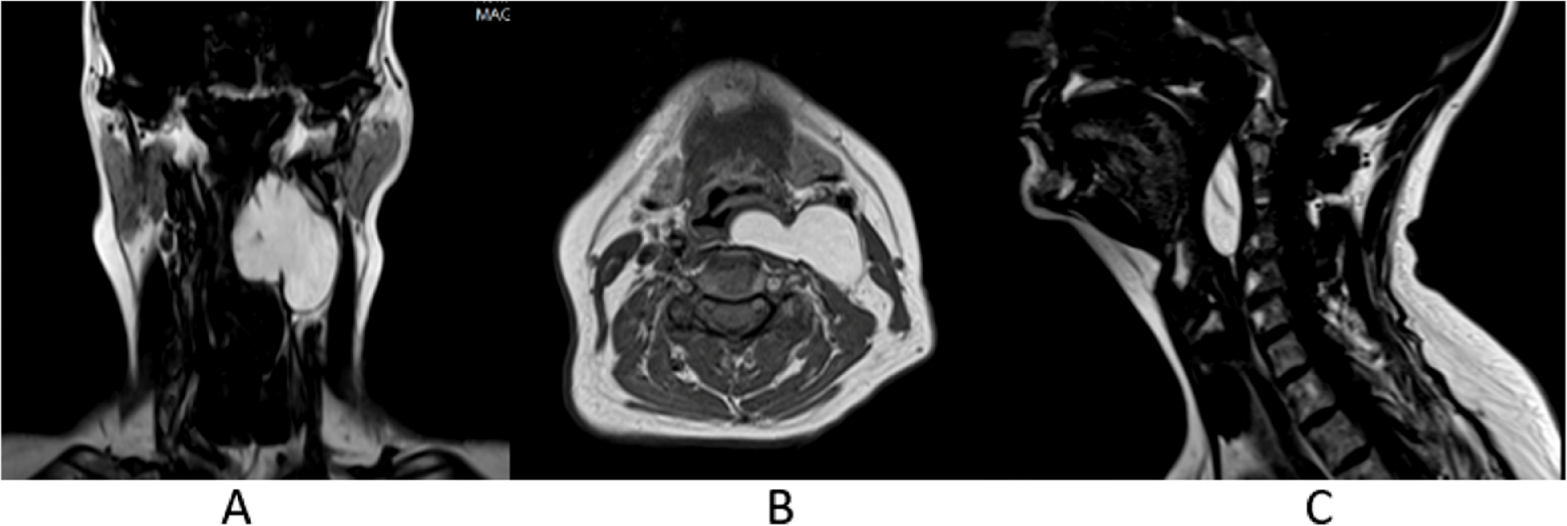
MRI T1-weighted coronal (a), axial (b), and sagittal (c) images showing strong enhancement of the lesion. Impingement on the airway as well as evidence of internal septation can be readily seen
